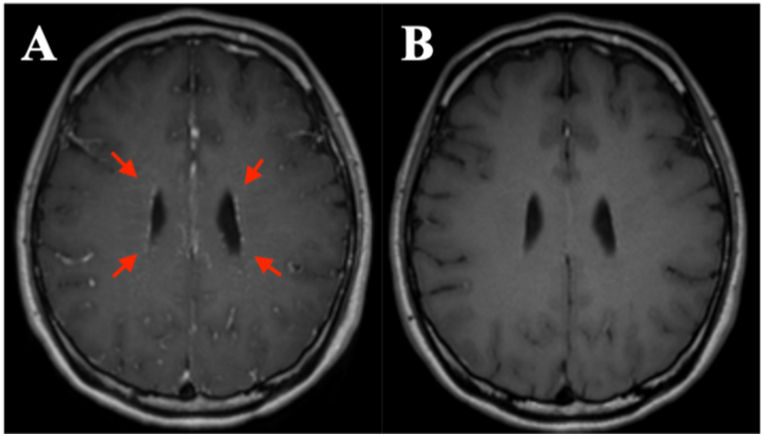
MRI before and after treatment A) Pre-treatment images. Contrast-enhanced T_1_-weighted MRI scan of the head shows a linear contrast effect perpendicular to the lateral ventricular wall and along the perivascular vessels spreading radially (red arrows). Anti-GFAPα antibody was detected in the cerebrospinal fluid of the patient and a diagnosis of GFAP-A was made. Half of the patients with GFAP-A show this sign [4]. B) Post-treatment images. The patient was treated symptomatically. Loxoprofen 60 mg or acetaminophen 400 mg was prescribed as needed (pro re nata), depending on the pain. A linear contrast effect that was present before treatment has disappeared. MRI, magnetic resonance imaging GFAP-A, glial fibrillary acidic protein astrocytopathy.
